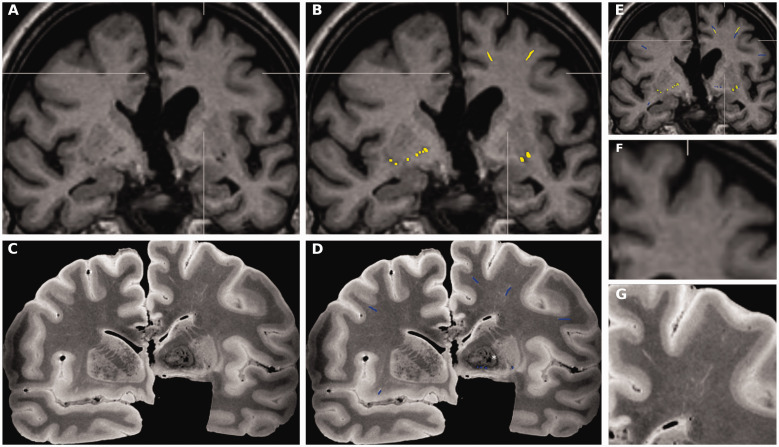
VRS *in vivo* vs. post-mortem quantification. All images in Figure 1 are taken from a female subject (#125-19), 67-years of age, who died 17 days after the MRI acquisition (see Table 1 for details). (a) On *in vivo*T1-weighted coronal images, two VRS are depicted in the medial/superior frontal gyrus and middle frontal gyrus in the left hemisphere (detailed in insert f) and bilaterally at the level of the perforating lenticulostriatal arteries. (b) VRS were manually segmented on *in vivo* images in the entire brain without knowledge of the post-mortem images (yellow color). (c) On T2-weighted images from the corresponding post-mortem sections, additional VRS are visualized in the right middle frontal gyrus (detailed in insert g), right insula, and left inferior frontal gyrus. (d) VRS were manually segmented on the entire T2 weighted images, without knowledge of the *in vivo* images. (c and d) Areas of low signal intensity are noted in the left globus pallidum (in the right side of the image, indicated with *), findings are consistent with idiopathic calcium depositions, a frequent incidental finding in elderly subjects. (e) After manual registration/alignment of post-mortem with *in vivo* images, VRS segmentations from both sessions were displayed on top of the *in vivo* scan and the number of *in vivo* VRS with the corresponding post-mortem VRS were determined at the section level with the highest anatomical alignment. (f) *In vivo* T1-weighted coronal images, magnified from (a). (g) Post-mortem T2-weighted coronal images, magnified from (c). VRS: Virchow-Robin Spaces.
